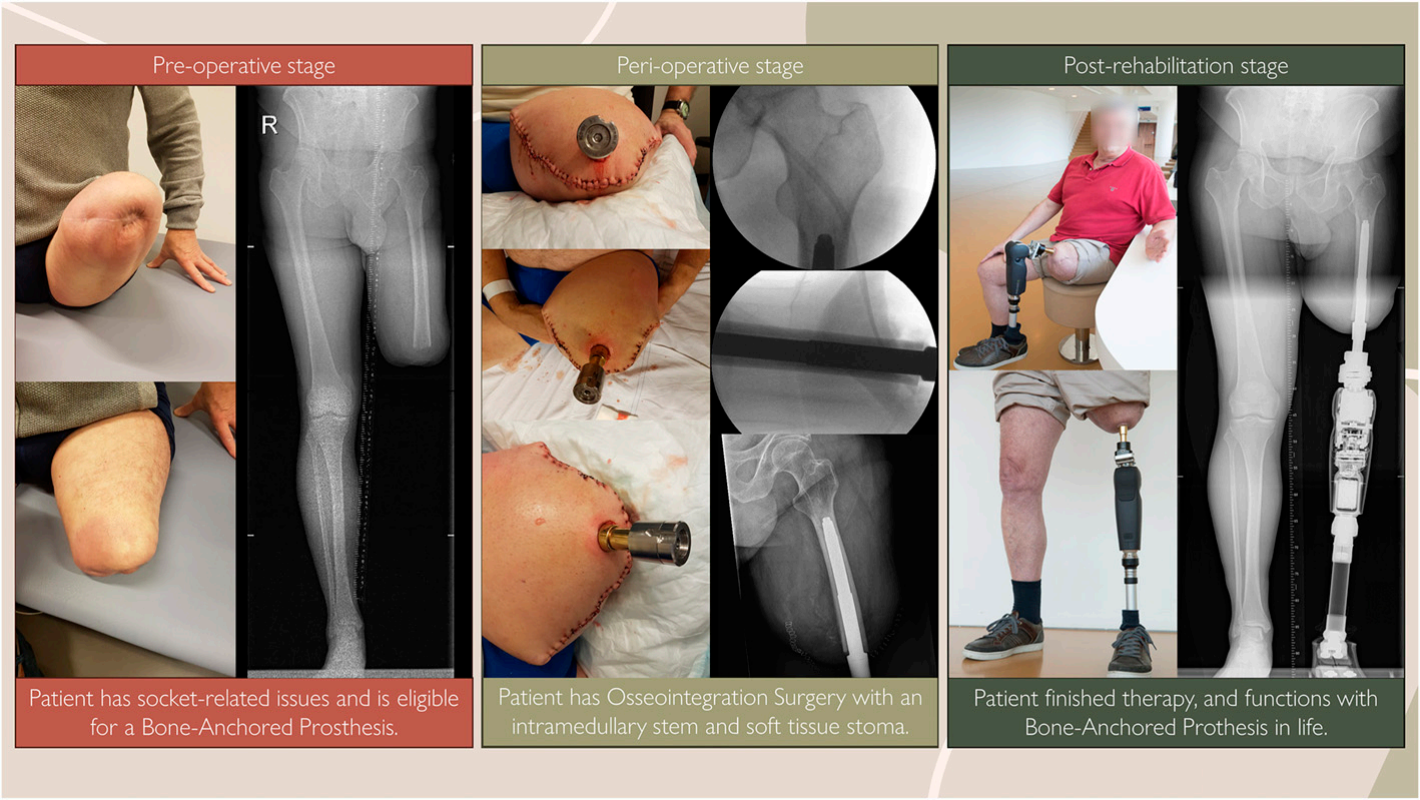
Different stages of bone-anchored prosthesis treatment over time and as shown on radiographic imaging.
